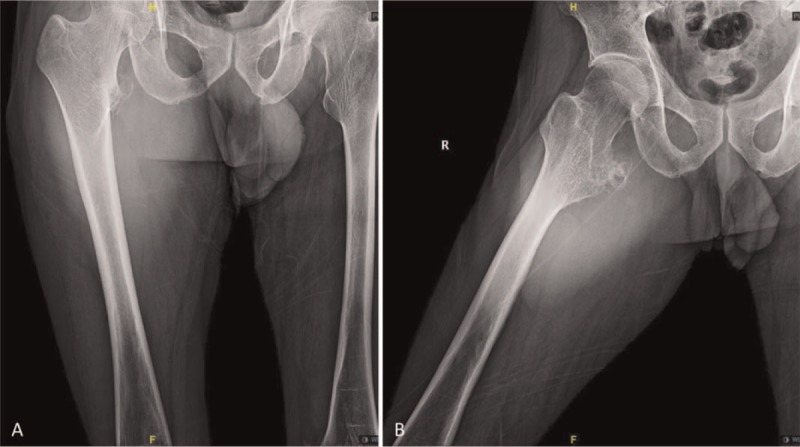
(A, B) Posteroanterior X-ray film of the right hip revealed irregular shadow of a soft tissue mass in the right thigh.
